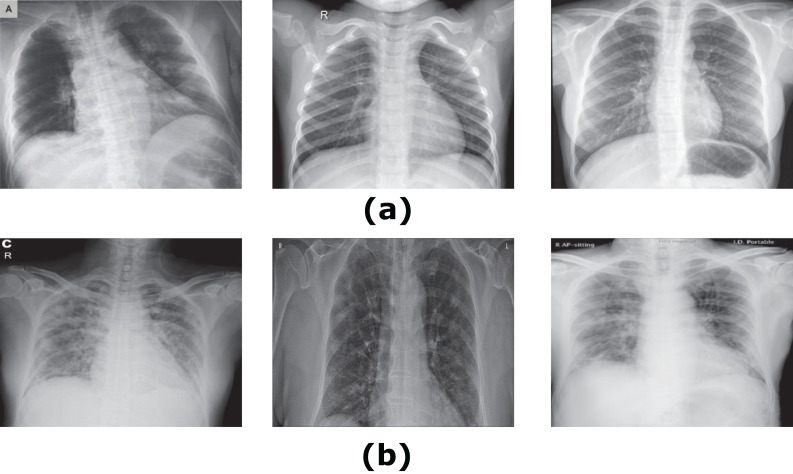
Samples of Dataset-1 (Faizan, 2020). (A) Normal X-Ray images, (B) infected COVID-19 X-Ray images. The CXR images were downloaded from GitHub under a CC0 1.0 license.
